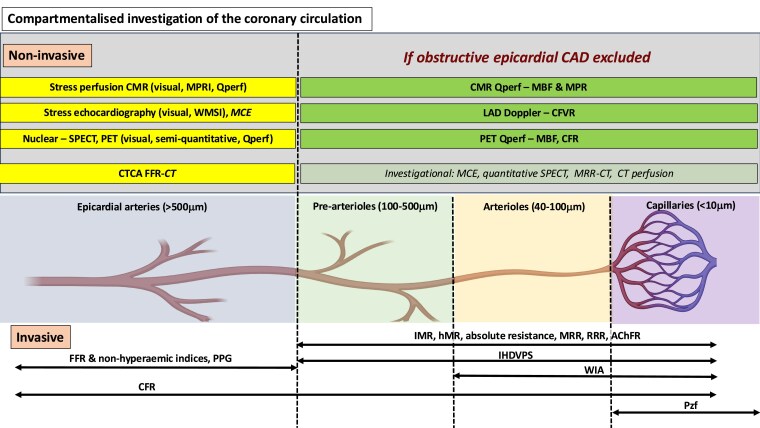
A compartmentalized approach to the non-invasive and invasive investigation of the coronary circulation to identify myocardial ischaemia. AChFR, acetylcholine flow reserve; CFR, coronary flow reserve; CFVR, coronary flow velocity reserve; CMR, cardiac magnetic resonance imaging; CT, computed tomography; CTCA, computed tomography coronary angiography; FFR, fractional flow reserve; FFR-CT, fractional flow reserve computed tomography; hMR, hyperaemic microvascular resistance; IHDVPS, instantaneous hyperaemic diastolic velocity-pressure slope index; IMR, index of microvascular resistance; LAD, left anterior descending artery; MBF, myocardial blood flow; MCE, myocardial contrast echocardiography; MRR, microvascular resistance reserve; MRR-CT, microvascular resistance reserve computed tomography; MPR, myocardial perfusion reserve; MPRI, myocardial perfusion reserve index; PET, positron emission tomography; Pzf, pressure at zero flow; PPG, pullback pressure gradient; Qperf, quantitative perfusion; RRR, resistance reserve ratio; SPECT, single-photon emission computed tomography; WIA, wave intensity analysis; WMSI, wall motion score index
